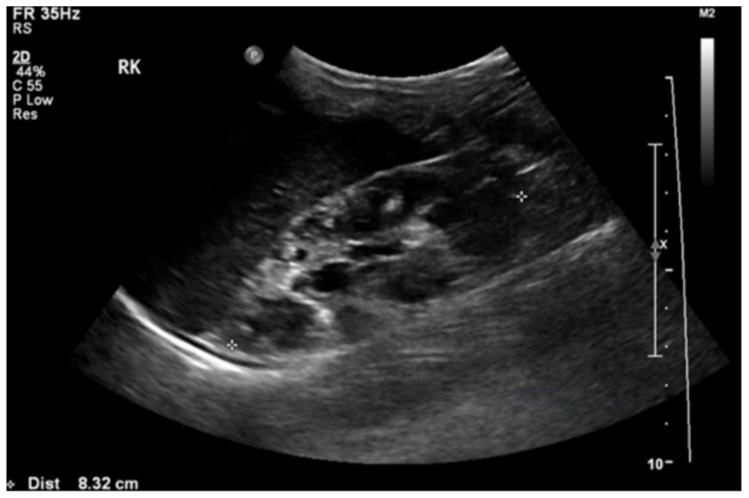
Renal ultrasound showed loss of corticomedullary differentiation of the right kidney with multiple cysts of variable sizes.
